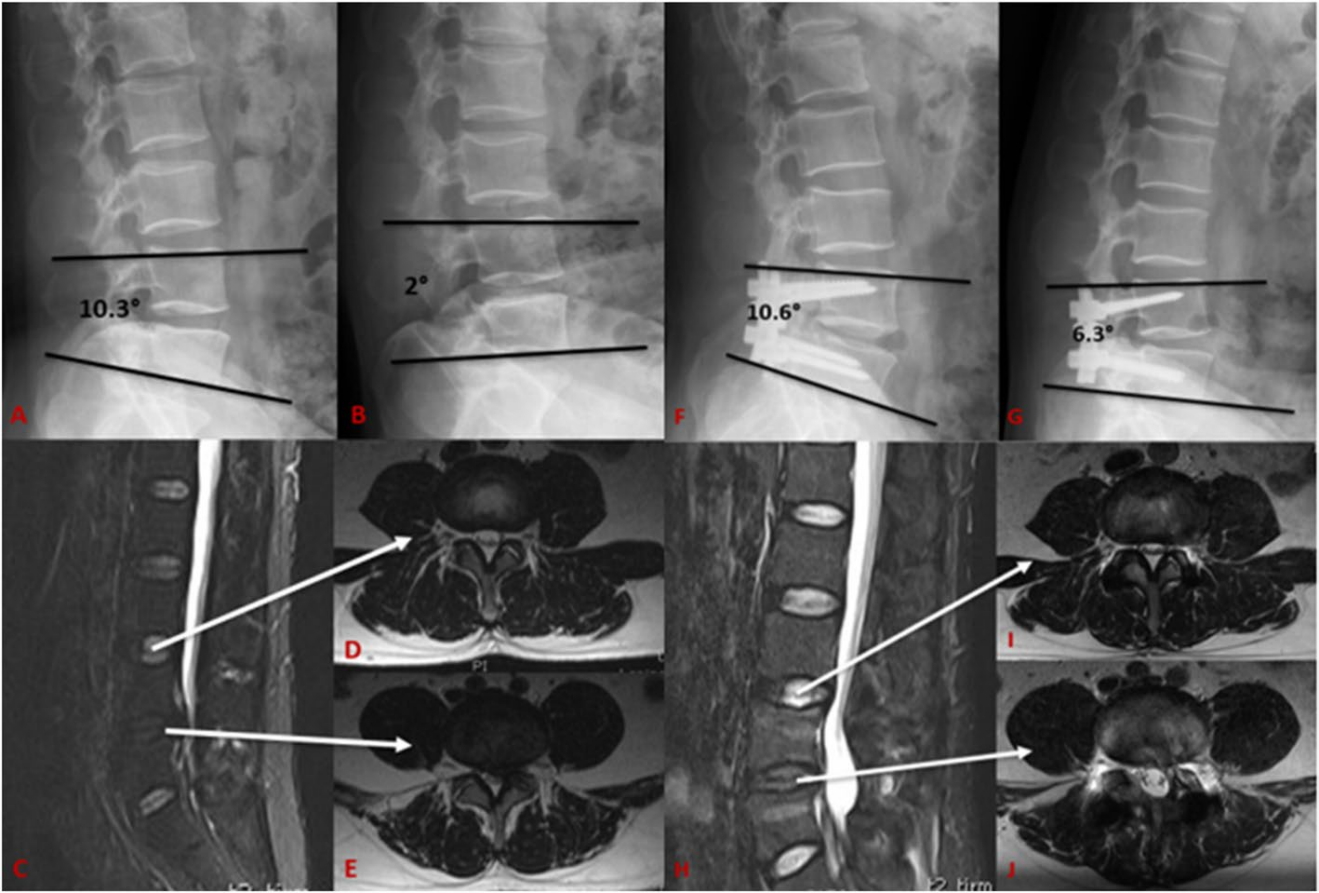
A 55-year-old man had spinal stenosis and disc herniation at L3–4, and the ROM of the surgical segment was 8.3° based on X-ray (A-B). The MRI of pre-operation showed that the disc signal of L3/4 and L4/5 (C-E). He underwent fenestration decompression and posterior EVO dynamic fixation at L4–5. The radiographs obtained 53 months after the operation showed the operative segment’s ROM was 4.3°, and the ISH of the L4/L5 remained unchanged from preoperative. The L3/L4 and L4/5 intervertebral disc signal was normal, with no significant change from preoperative (F-J)
